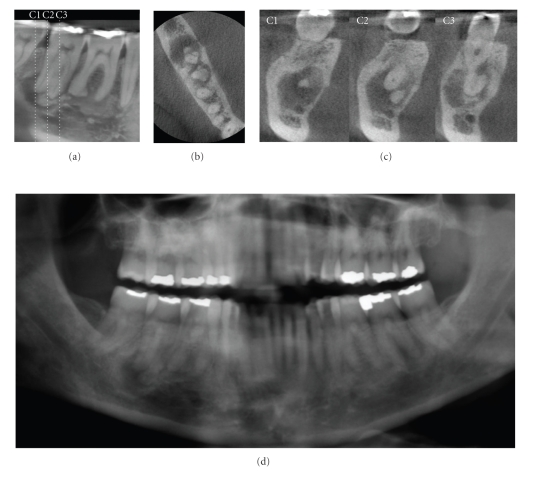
A 52-year-old Caucasian female was referred for assessment of multiple periapical areas associated with the mandibular right first and second molars. Curved planar (a), axial (b), and correlated multiple cross-sectional (c) images are shown. There are areas of mixed central opacity and peripheral radiolucency associated with the apices of the teeth; however no expansion, tooth resorption, or displacement is evident. Hypercementosis is observed on the distal root of the first molar. On clinical examination, all teeth in this quadrant tested vital. Based on a working diagnosis of florid cemento-osseous dysplasia, an additional digital panoramic radiograph ((d) cropped panoramic image) was performed and revealed similar bony patterns in the left posterior maxilla and mandible. Management of this patient comprised a 6-month recall comparison of focused CBCT images to judge the progression of the lesion.
